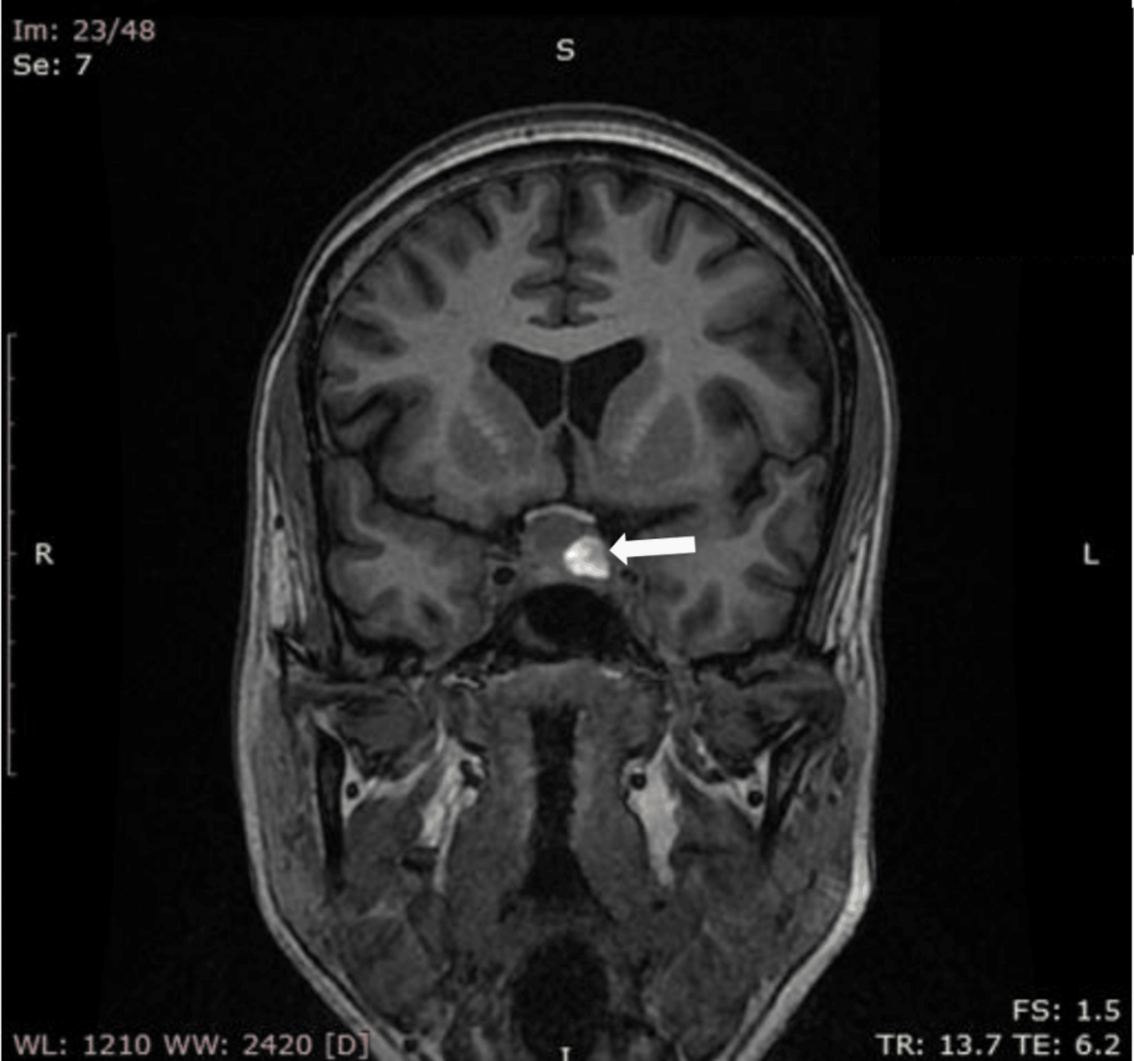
Case 3: Coronal T1-weighted MRI (SPGR sequence) shows a hypointense pituitary mass (macroadenoma) of size 1.1 cm x 1.5 cm x 1.6 cm (anteroposterior x transverse x craniocaudal) with a hyperintense focus (arrow) along the left lateral aspect that represents intratumoral haemorrhage. Optic chiasmal compression is also evident. SPGR: spoiled gradient recalled
